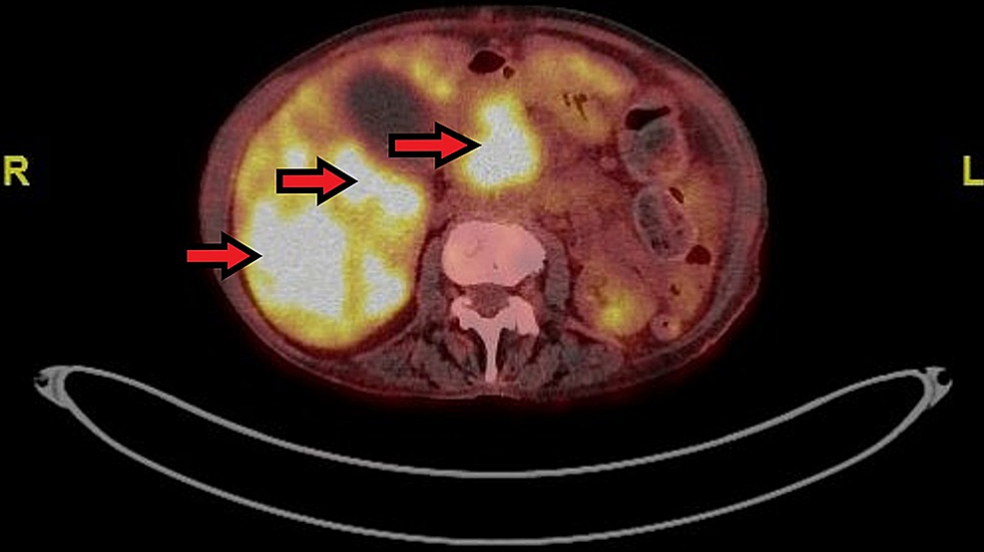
Transverse plane view of patient’s Ga68-DOTATATE-PET/CT scan demonstrating multiple foci of intense increased tracer uptake within both lobes of the liver and within the proximal pancreas, signifying a metastatic NET NET - neuroendocrine tumour
